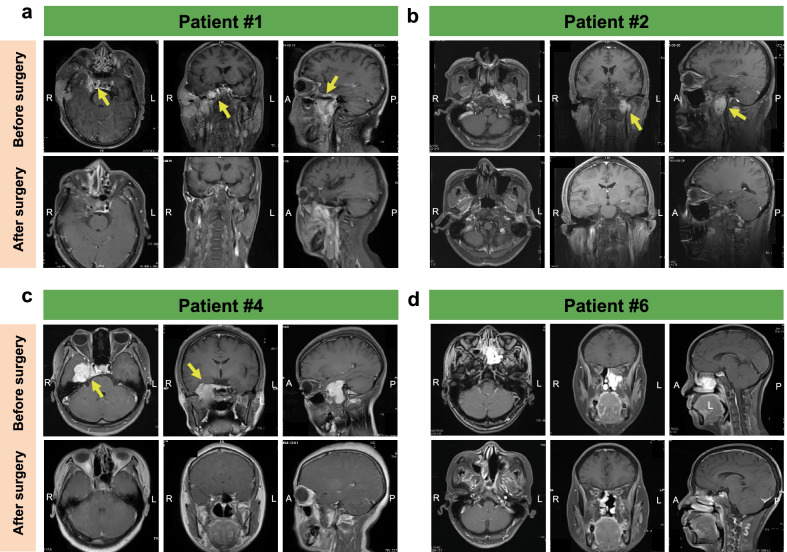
Preoperative and postoperative MRI images of the cranial base. a Patient #1. b Patient #2. c Patient #4. d Patient #6
